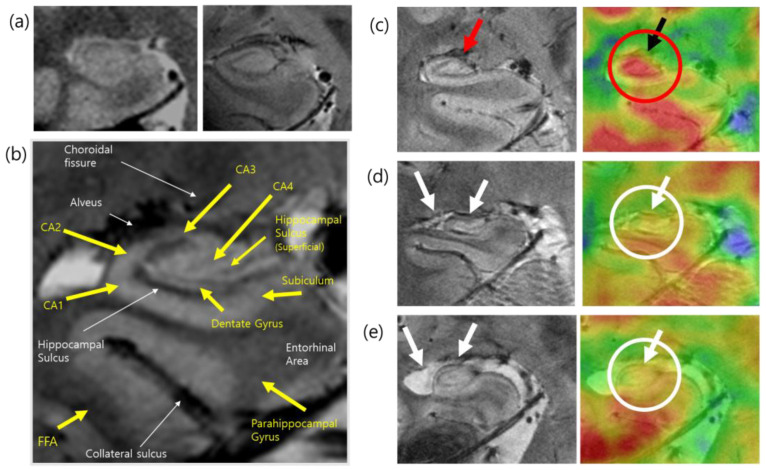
(a) Comparison of hippocampus imaging in 1.5T (left) and 7.0T (right) MRI. (b) Anatomical labeling of the hippocampus substructure in the 7.0T MR image. This research was originally published in JNM [52]. © SNMMI. (c) A 7.0T structural MRI and corresponding [^18^F]FDG PET–MRI fusion image of the hippocampus of healthy individuals (d) and (e) hippocampal atrophy and deformation in the 7.0T MR images and the corresponding glucose metabolism in the [^18^F]FDG PET–MRI of patients with AD. FFA; fusiform face areas, CA1-4; cornu ammonis 1-4. Reprinted with permission from Cho et al., 2014, Elsevier [13].
